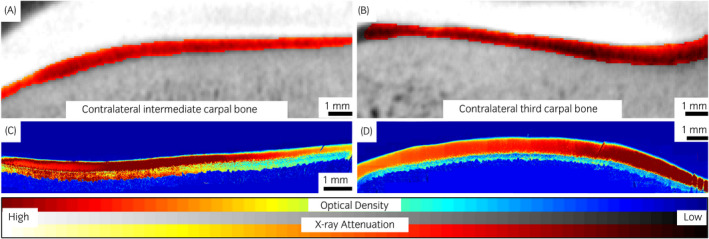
Computed tomography (A and B) and optical density maps (C and D) for contralateral (control) joints with intact articular cartilage surfaces of the third carpal bone and the intermediate carpal bone for two individual ponies. Computed tomography imaging was conducted at 60 minutes after the contrast agent injection procedure
